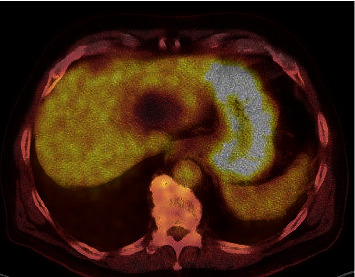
Axial CT fused image of FDG PET examination demonstrating no evidence of abnormal metabolic activity within segment 4 hepatic lesion.
